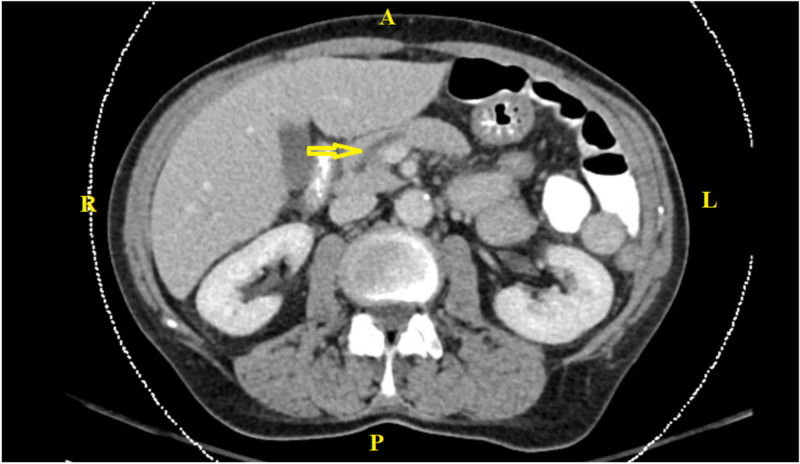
CT of the abdomen and pelvis with contrast The image shows subtle fat stranding along the pancreatic head and slightly prominent pancreatic duct, which can be seen with early interstitial edematous pancreatitis (arrow) CT: computed tomography
